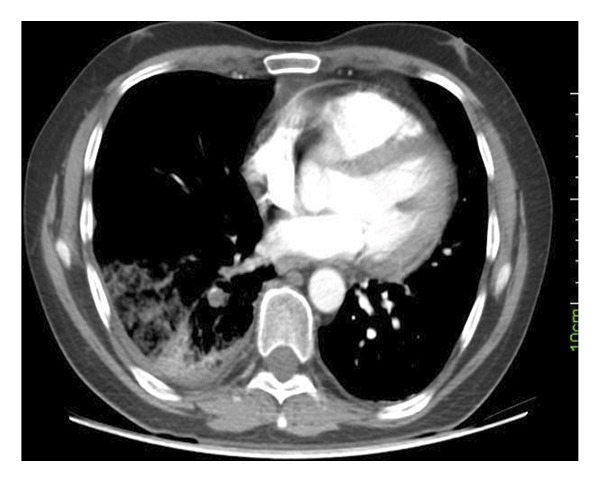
Axial CT image showing wedge‐shaped peripheral consolidation in the right lower lobe, suggestive of pulmonary infarction. Filling defect in the right lower lobe pulmonary artery is clearly visible.
